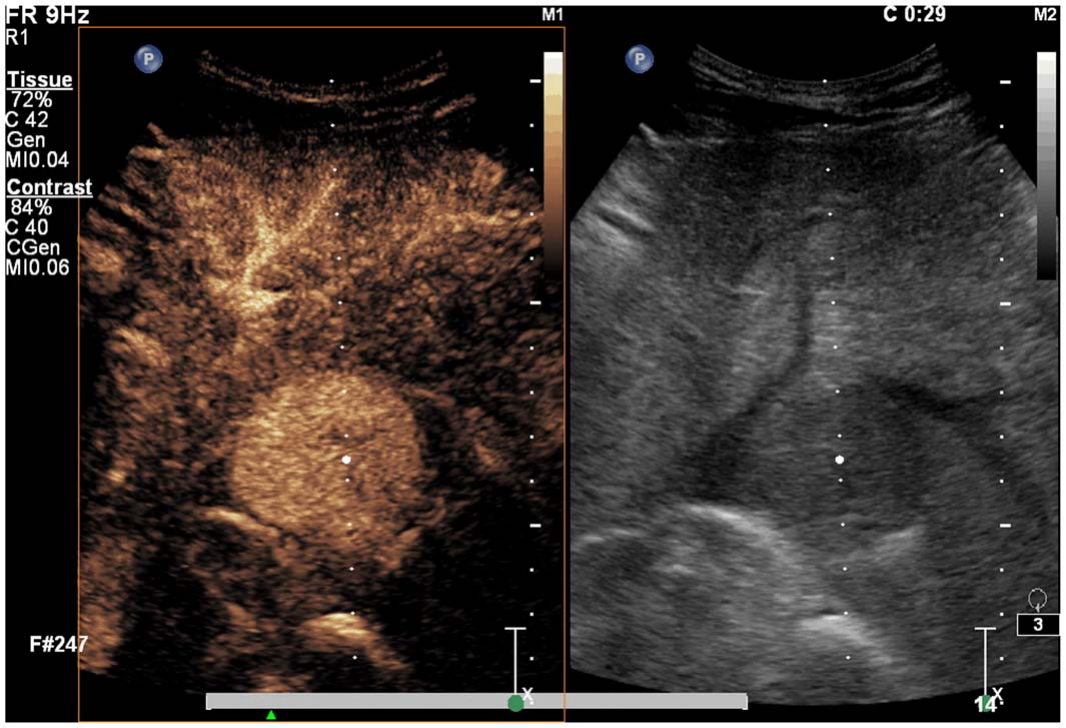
A spherical lesion with enhanced blood flow. Before treatment, a spherical lesion and enhanced blood flow is shown by contrast ultrasound.
